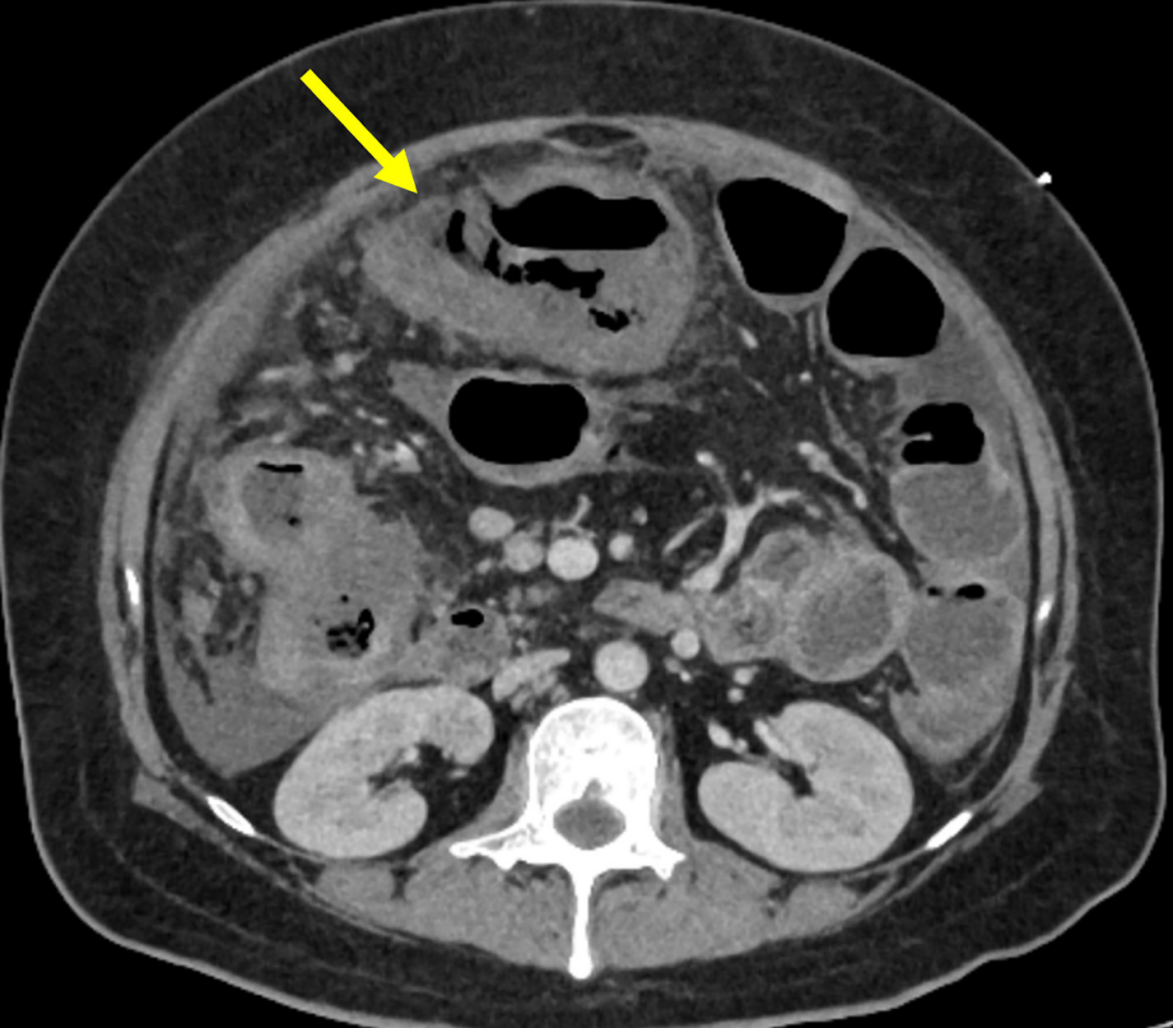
Abdominal CT on admission. The wall of the transverse colon thickened (yellow arrow), and the density of the surrounding fat tissue increased. A reduction in contrast enhancement was observed in parts of the transverse colonic wall.
